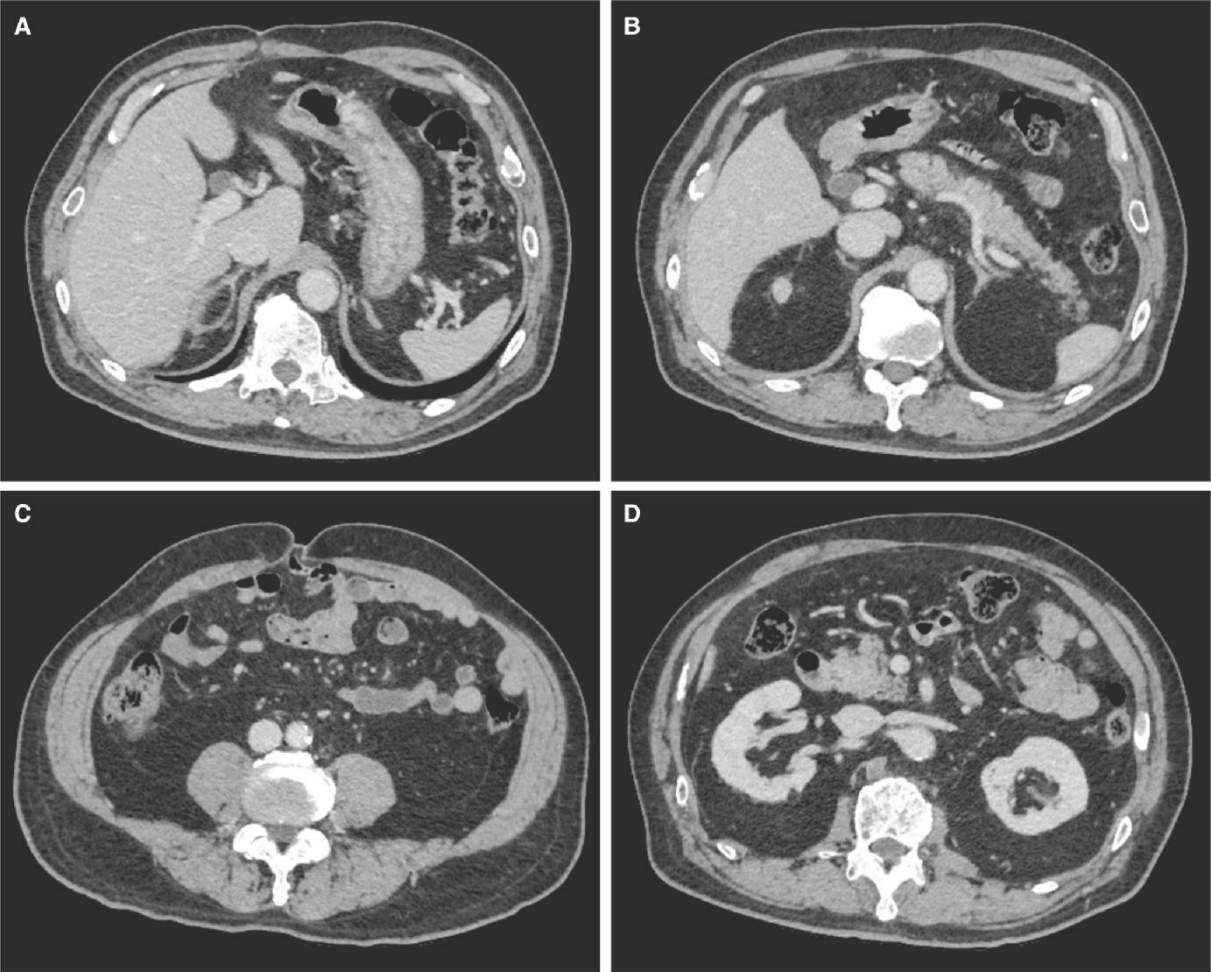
Computed tomography examination. No definite adrenal nodule was found in the bilateral adrenal glands. (A) Right adrenal gland. (B) Left adrenal gland. (C) Visceral adipose tissue accumulation was noted compared with the subcutaneous adipose tissue. High accumulation of visceral fat (145.4 cm^2^) and a visceral/subcutaneous adipose tissue ratio of 0.53 (reference range: <0.4) were observed. (D) Thinned bilateral cortexes of kidneys are presented.
